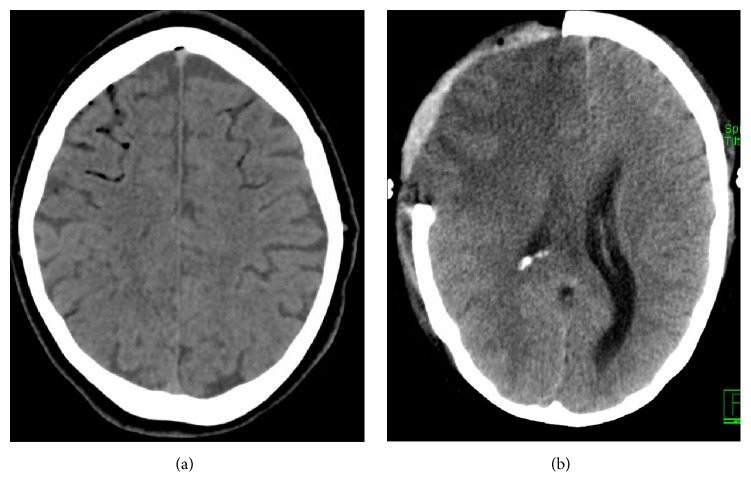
(a) Emergency noncontrast CT scan demonstrating air emboli affecting frontal lobes bilaterally. (b) Repeat CT scan at day four following decompressive hemicraniectomy for consequent infarction.
